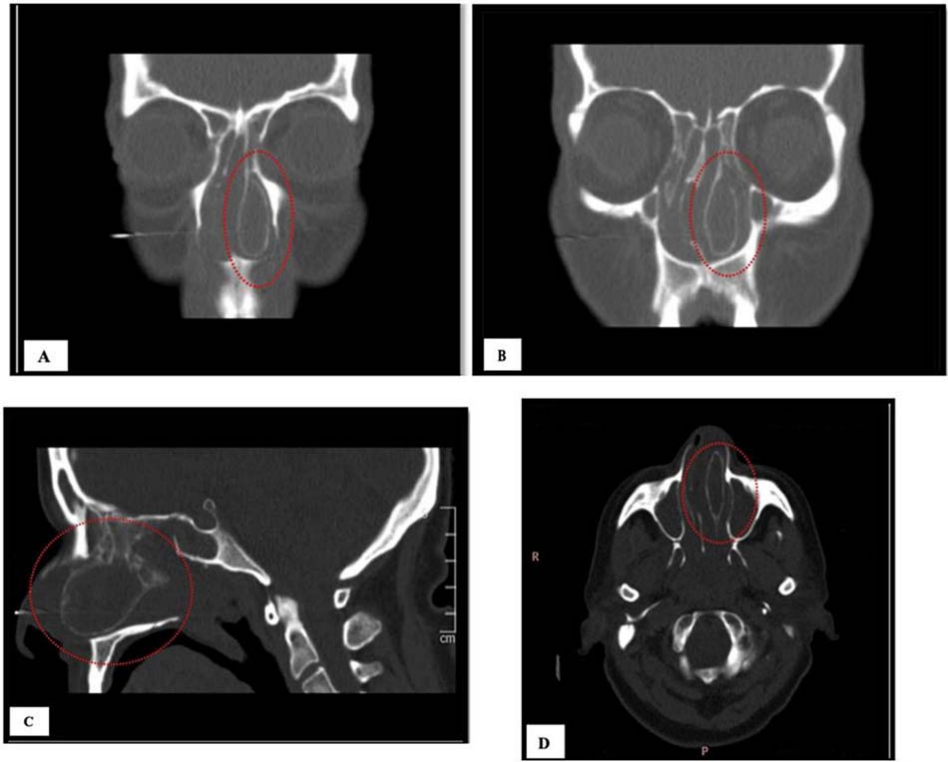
(A, B) CT of coronal view; (C) CT of sagittal view, and (D) CT of axial view.
